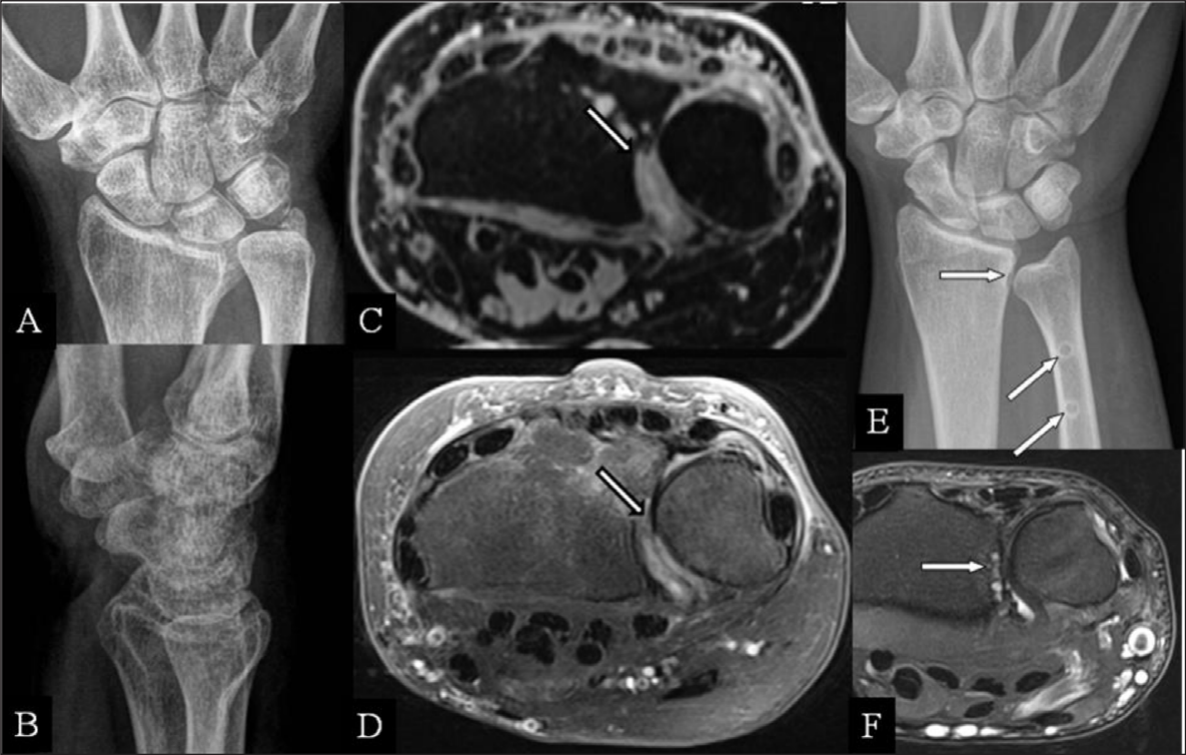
Radioulnar abutment and ulnar impingement. (A, B) PA and lateral plain radiographs; (C) Axial 3D-GRE; (D) SE T2-WI FS; (E) PA plain radiograph; and (F) Axial SE T2-WI FS. (A, B) Sequelae of a Pouteau-Colles fracture of the distal radial epiphysis. (C) Residual step-off at the radial sigmoid notch. (D) Destruction of the cartilage at the dorsal part of the sigmoid notch. (E) Excessive shortening (horizontal arrow) after surgery (oblique arrows). (F) subchondral erosions at the most proximal part of the radial sigmoid notch.
